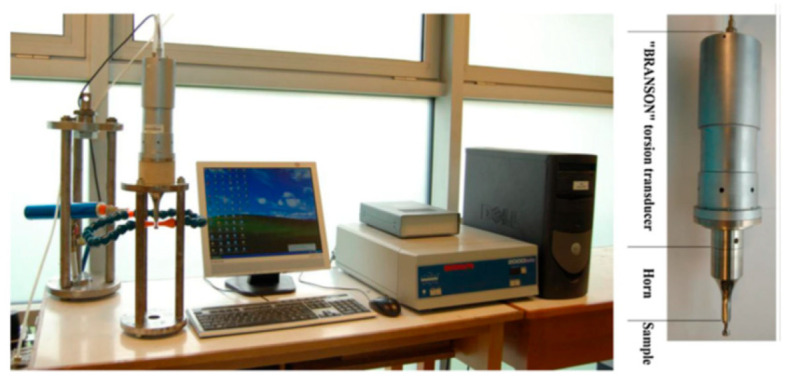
Photos of the new ultrasonic torsion testing system [42].
